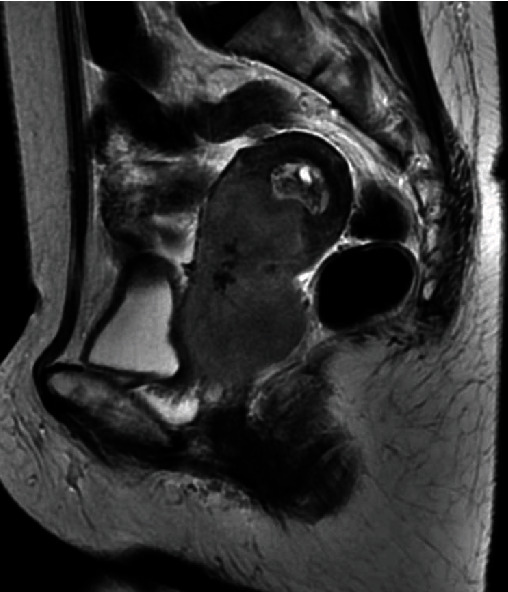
Magnetic resonance imaging (MRI) findings of primary disease. T2-weighted MRI showing locally advanced cervical cancer that extended into the endometrium.
